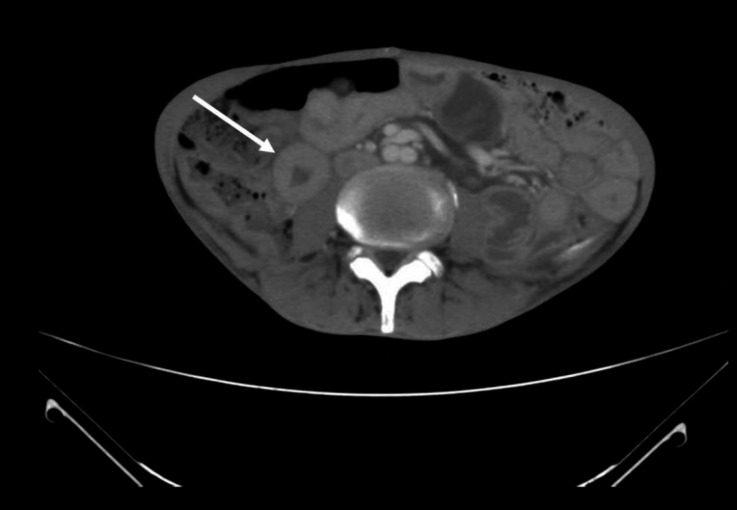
CT scan performed on February of 2021 and showed a questionable circumferential wall thickening (arrow) of a distal small bowel loop in the right side of the abdomen which needs further assessment
